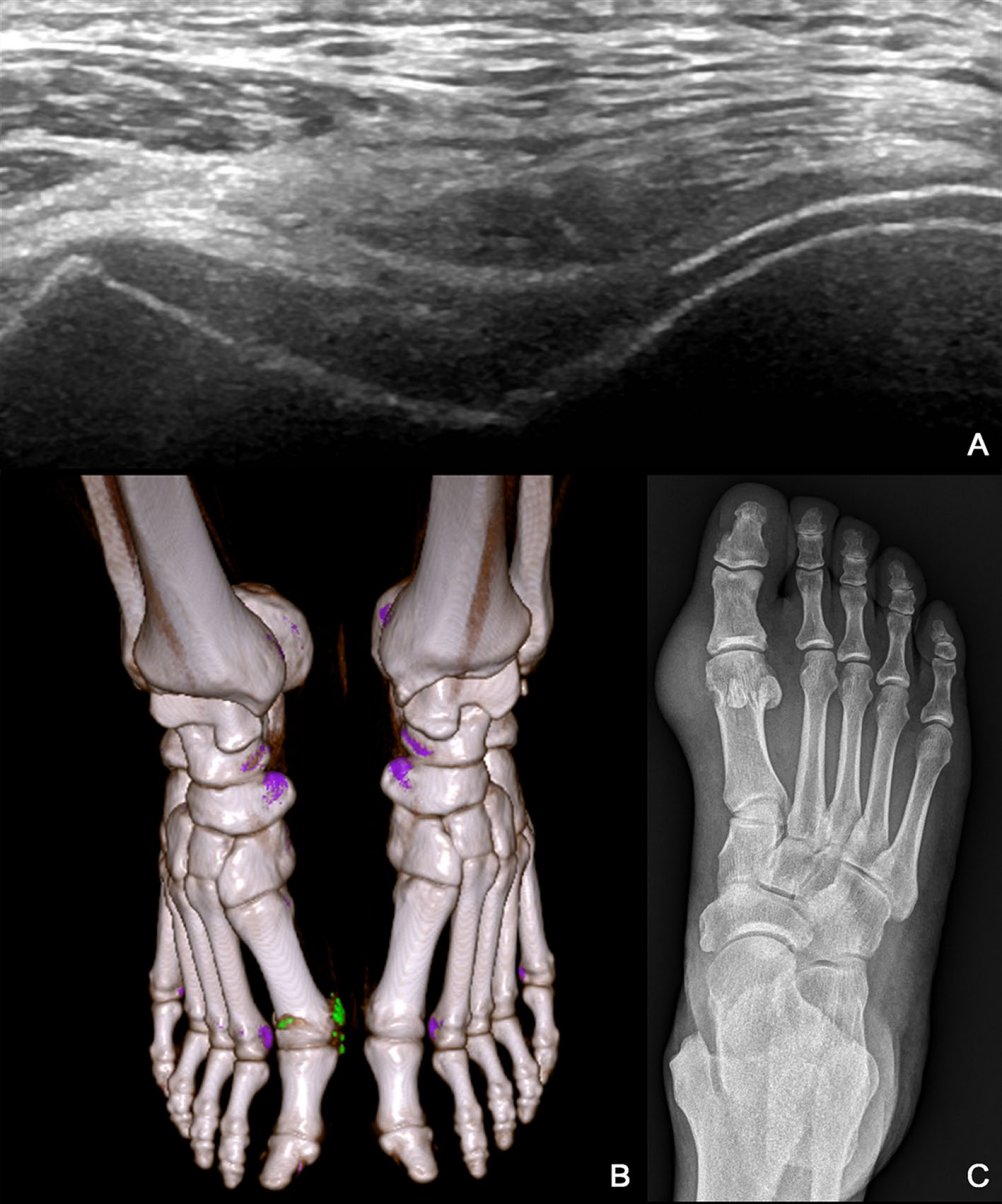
Imaging features included in the classification criteria: (A) Transverse ultrasonographic image showing double-contour in femoral articular cartilage. The image features hyperechoic enhancement over the surface of the hyaline cartilage; (B) Dual-energy computed tomography scan showing urate deposition at the right first metatarsophalangeal joint; (C) Conventional radiographic image showing signs of erosion in the first metatarsophalangeal joint, as indicated by a cortical break with sclerotic margin and overhanging edge.
